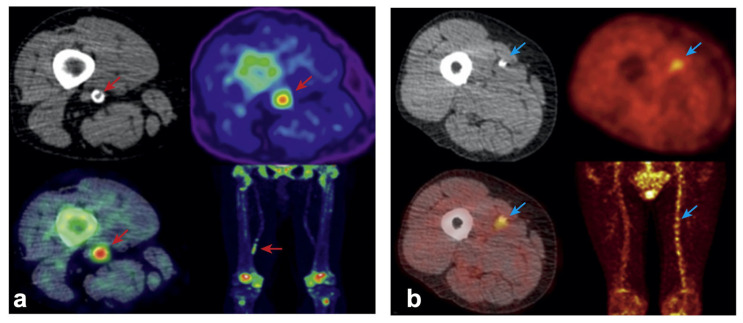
(a) Non-contrast CT (top left) with a rim of calcification of the vessel, ^18^F-NaF PET (top right), and fused ^18^F-NaF PET/CT (bottom left) of the superficial femoral artery (red arrow) at the level of the adductor canal, demonstrating significant vessel uptake in a patient with claudication at 300 yards. In addition, prominent uptake is seen in the vessel at the same level on the coronal image (bottom right); (b) non-contrast CT (top left) with calcification of the vessel, ^18^F-FDG PET (top right), and fused non-contrast CT (bottom left) of the superficial femoral artery (blue arrow) at the level mid-thigh, demonstrating significant vessel uptake in a patient with tissue loss in the left leg. In addition, prominent uptake is seen across the whole vessel coronal image (bottom right), compared with the contralateral leg. CT = computed tomography; ^18^F-FDG = ^18^F-fluorodeoxyglucose; ^18^F-NaF = ^18^F-sodium fluoride; PET = positron emission tomography. This image is reprinted from Chowdhury et al. with modification [106].
